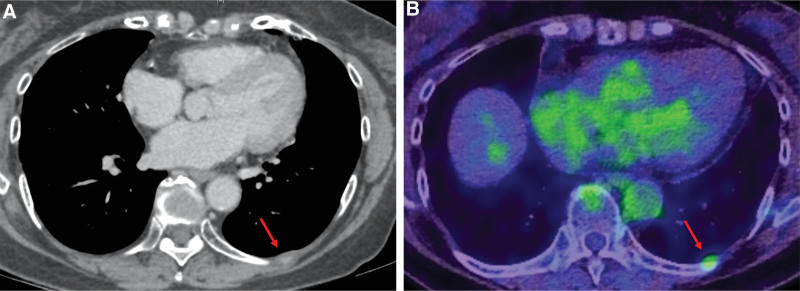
Computed tomography showed osteosclerotic changes (red arrow) in the left 9^th^ rib (A). This was diagnosed as recurrent solitary bone metastasis because positron emission tomography showed abnormal fluorine-18 fluorodeoxyglucose accumulation (red arrow) at the same site (B).
